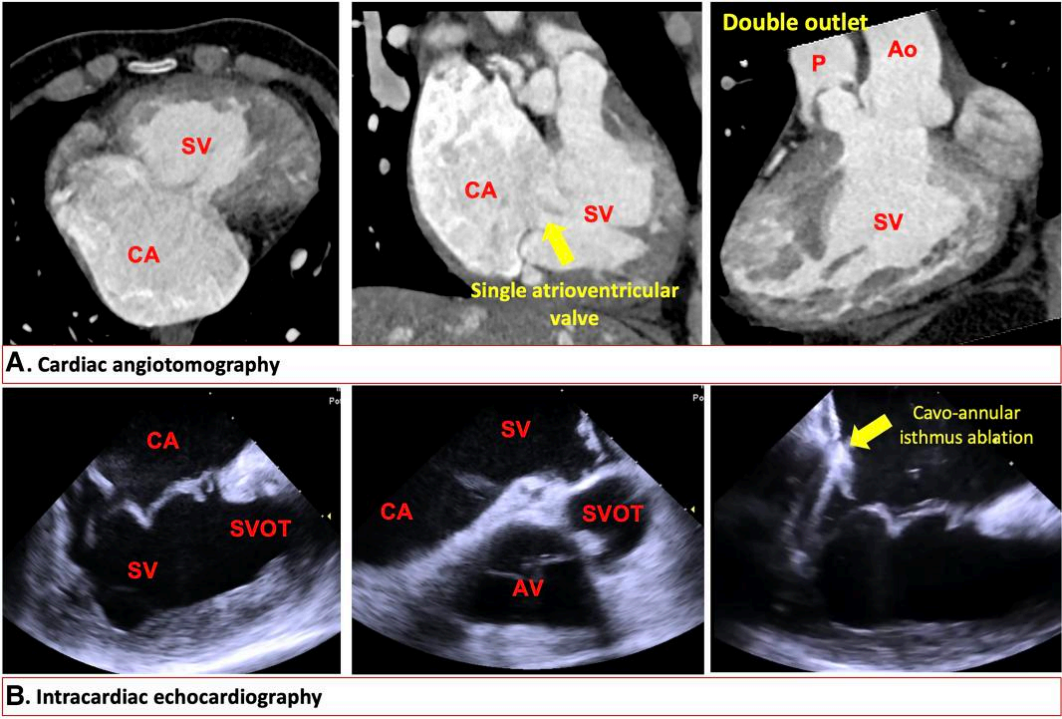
Cardiac computed tomography angiography and intracardiac echocardiography. (*A*) Cardiac computed tomography angiography reveals a morphologically right systemic single ventricle and a dilated common atrium, along with the absence of an interatrial septum and a single atrioventricular valve. The single ventricle exhibits a double-outlet configuration. (*B*) Intracardiac echocardiography. In the home view, the common atrium, single ventricle, and single atrioventricular valve are visualized. The moment of cavo-annular isthmus ablation guided by intracardiac echocardiography is also depicted. CA, common atrium; SV, single ventricle; P, pulmonary artery; Ao, ascending aorta; SVOT, single ventricle outflow tract; AV, aortic valve.
